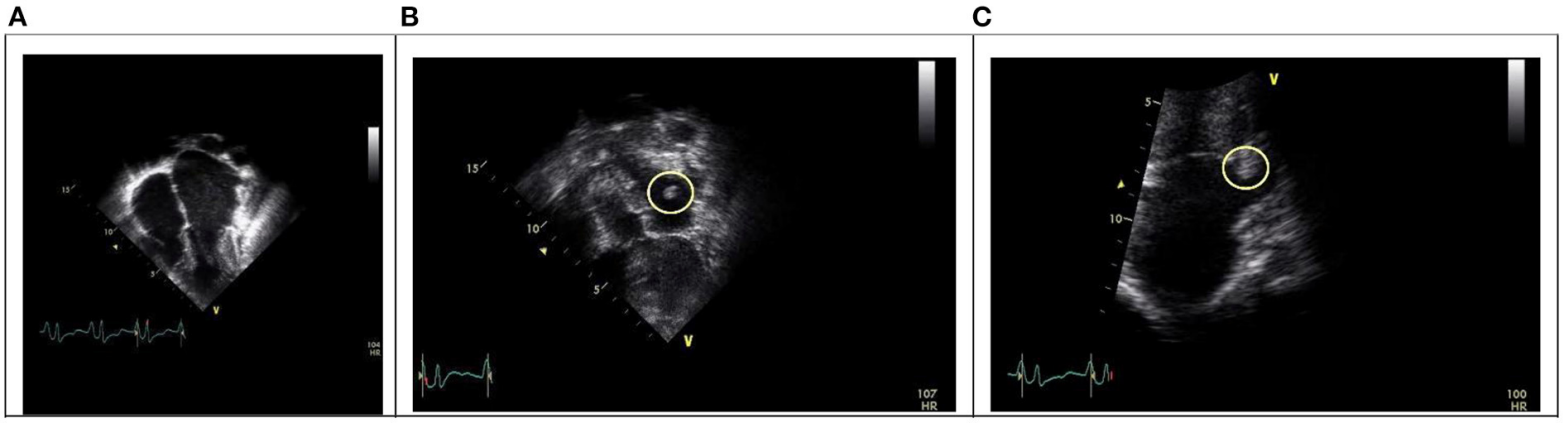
(A) Apical four chamber view showing bi-atrial dilatation with normal ventricles size. (B) A thrombus mass in the left atrium close to the left atrial appendage (yellow circle). (C) A thrombus mass in the left lower pulmonary vein.
